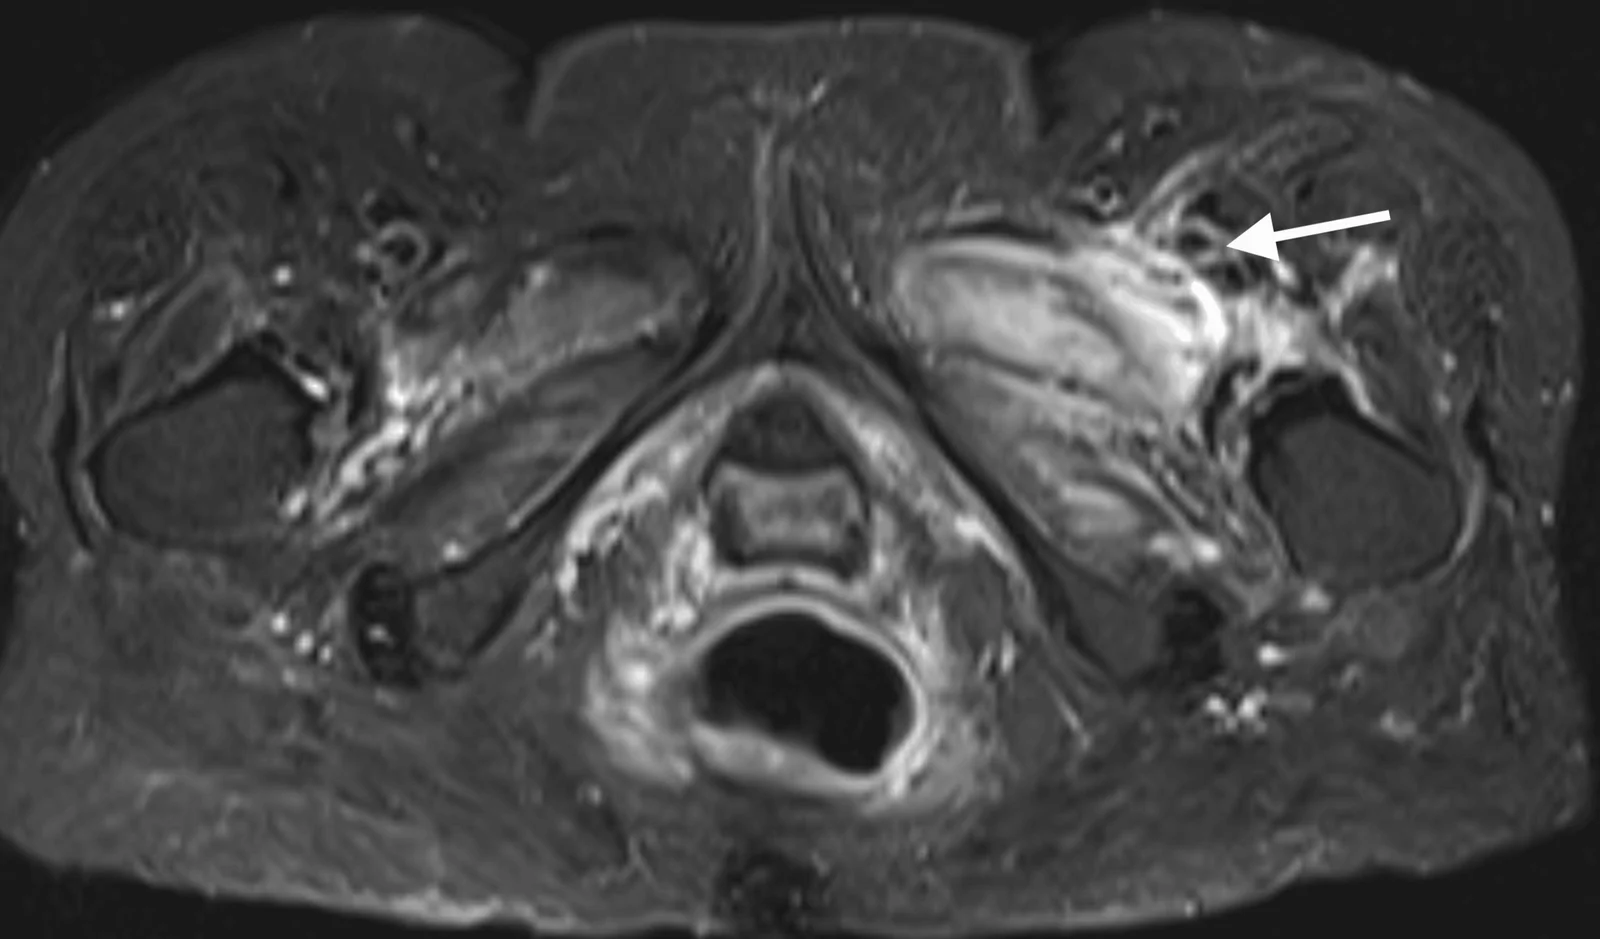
Axial fat-suppressed T2-weighted MRI of the pelvis showing left obturator internus myositis with abscess formation (arrow). The image shows hyperintense signal and enlargement of the left obturator internus muscle, with inflammatory changes in the adjacent fat, as indicated by the arrow. These findings are consistent with myositis with abscess formation.
